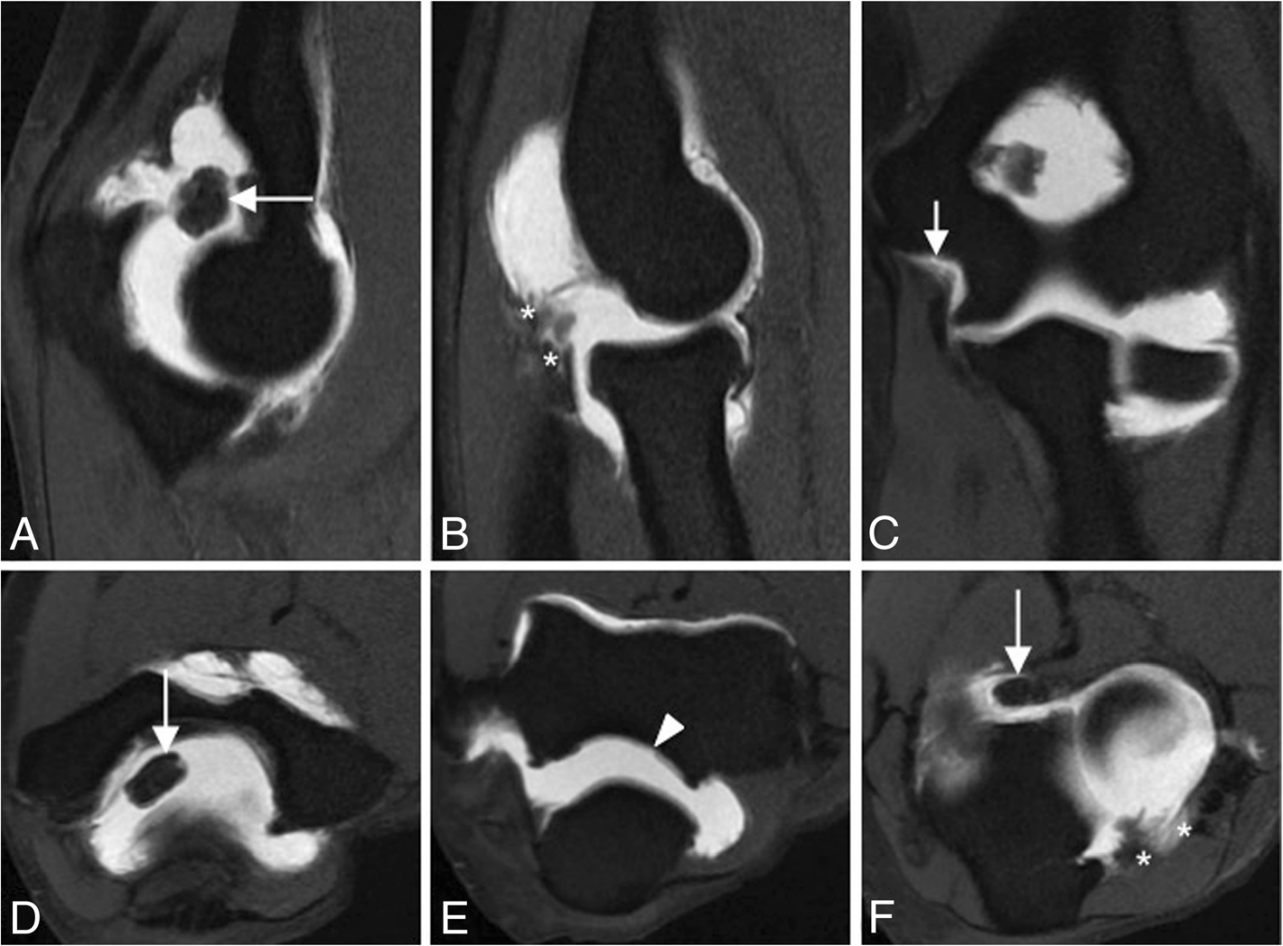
Sagittal FS T1-weighted direct MR arthrographic images (a, b), coronal FS T1-weighted direct MR arthrographic image (c), and axial FS T1-weighted direct MR arthrographic images (d–f) showing a proximal partial-thickness proximal tear of the anterior bundle of the medial collateral ligament complex (short arrow), disruption and stripping of the posterior and lateral capsular structures (white asterisks), intra-articular bodies (long arrows), and full-thickness defect at the posterior joint line (arrowhead). Note ulnohumeral incongruity (a) and radiocapitellar incongruity (b)
